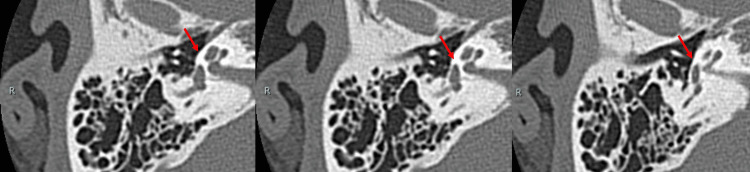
Axial HRCT images of the right temporal bone (Case 1) showing no sign of hypodense demineralized plaques in the region of the fissure ante fenestra (arrows). Three consecutive sections shown are 0.5 mm thick. HRCT, High-resolution computed tomography
